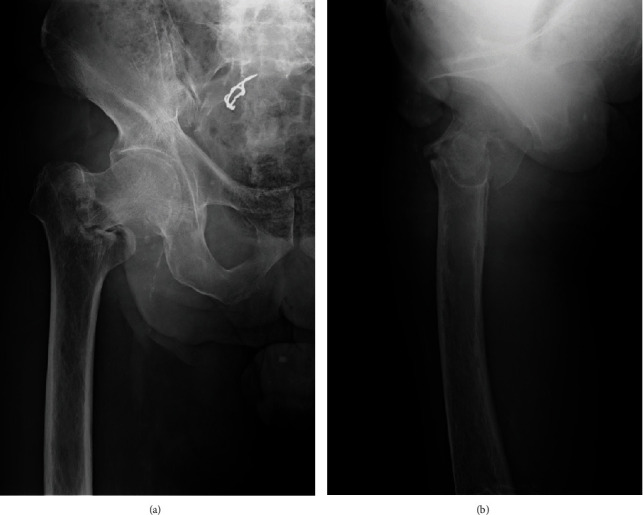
Anteroposterior (a) and lateral (b) radiographs taken on initial presentation showing a trochanteric fracture with a greater trochanteric fragment in the right femur, which are classified as AO type 31A2.2 and Jensen type 3.
